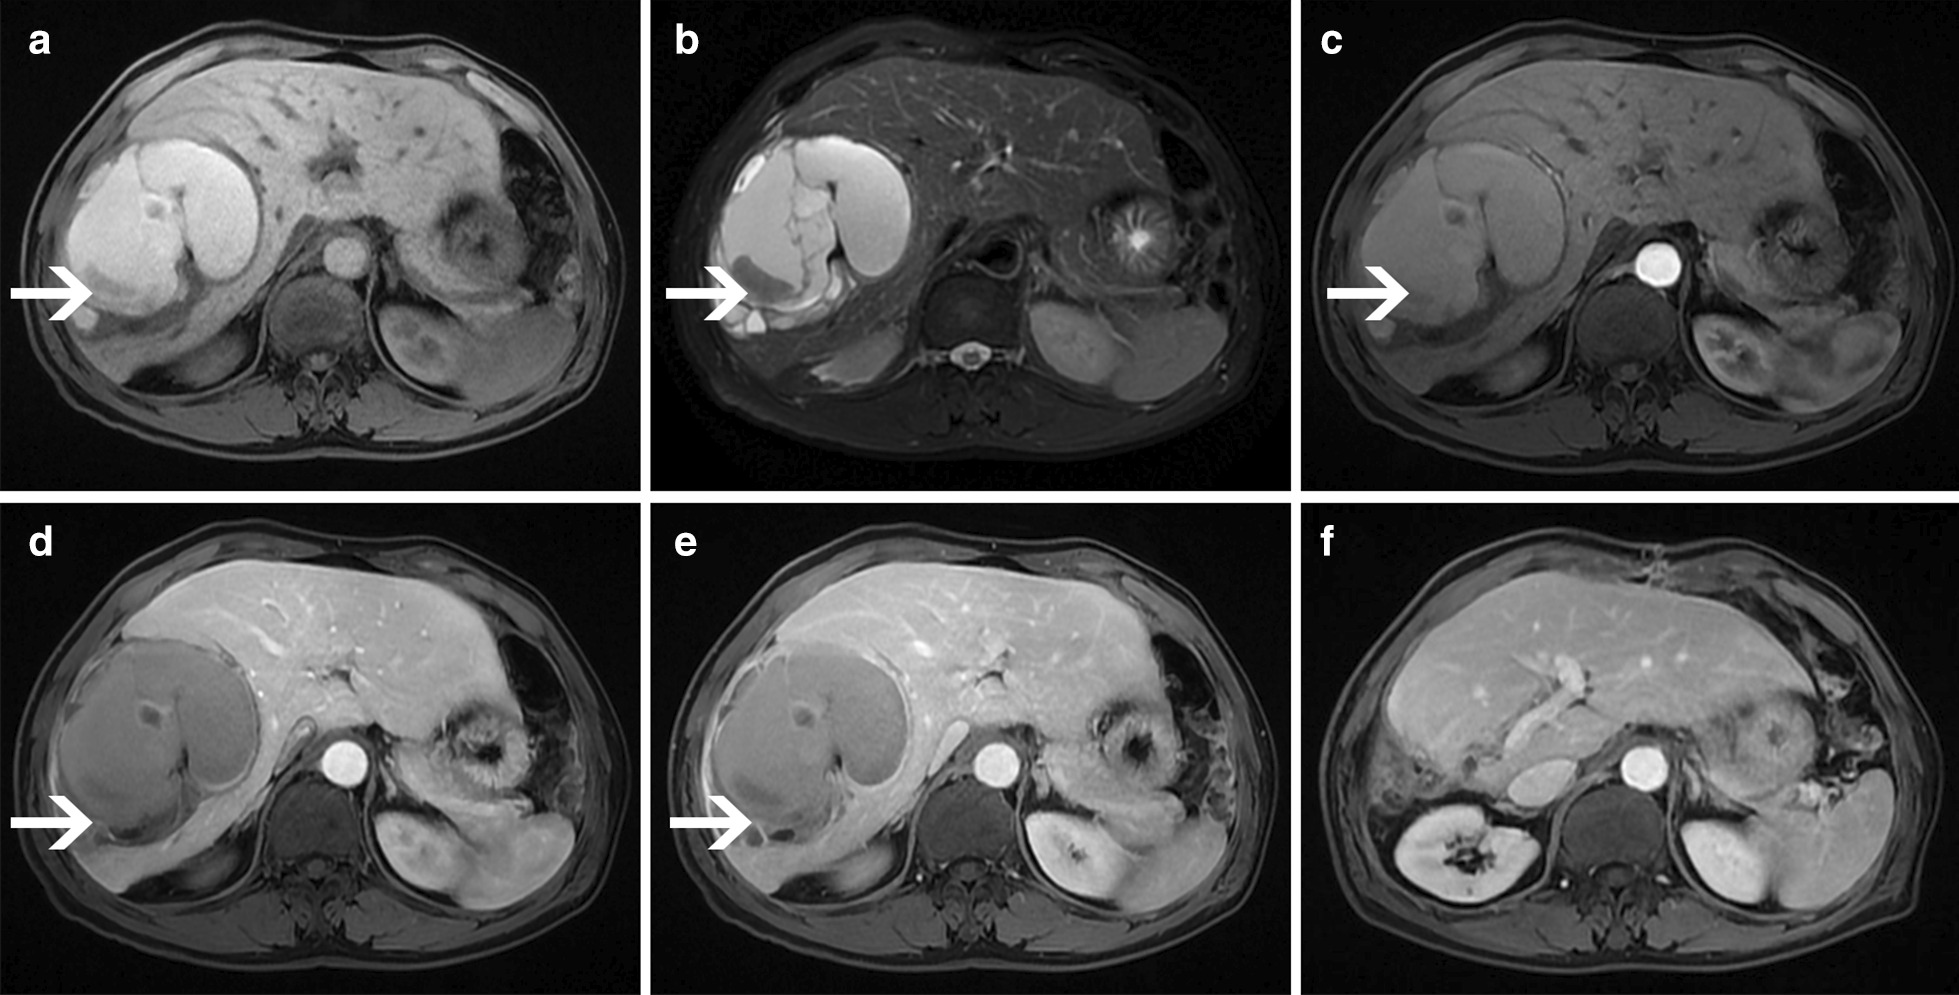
MRI examination. The lesion showed isointense or hyperintense signals on T1-weighted images (a) and T2-weighted images (b). Contrast-enhanced T1-weighted images showed no lesion enhancement: (c: arterial phase; d: venous phase; e: equilibrium phase). Contrast-enhanced T1-weighted images showed postoperative remnant liver volume increased significantly in the portal vein phase and no encapsulated peritoneal effusions (f)
